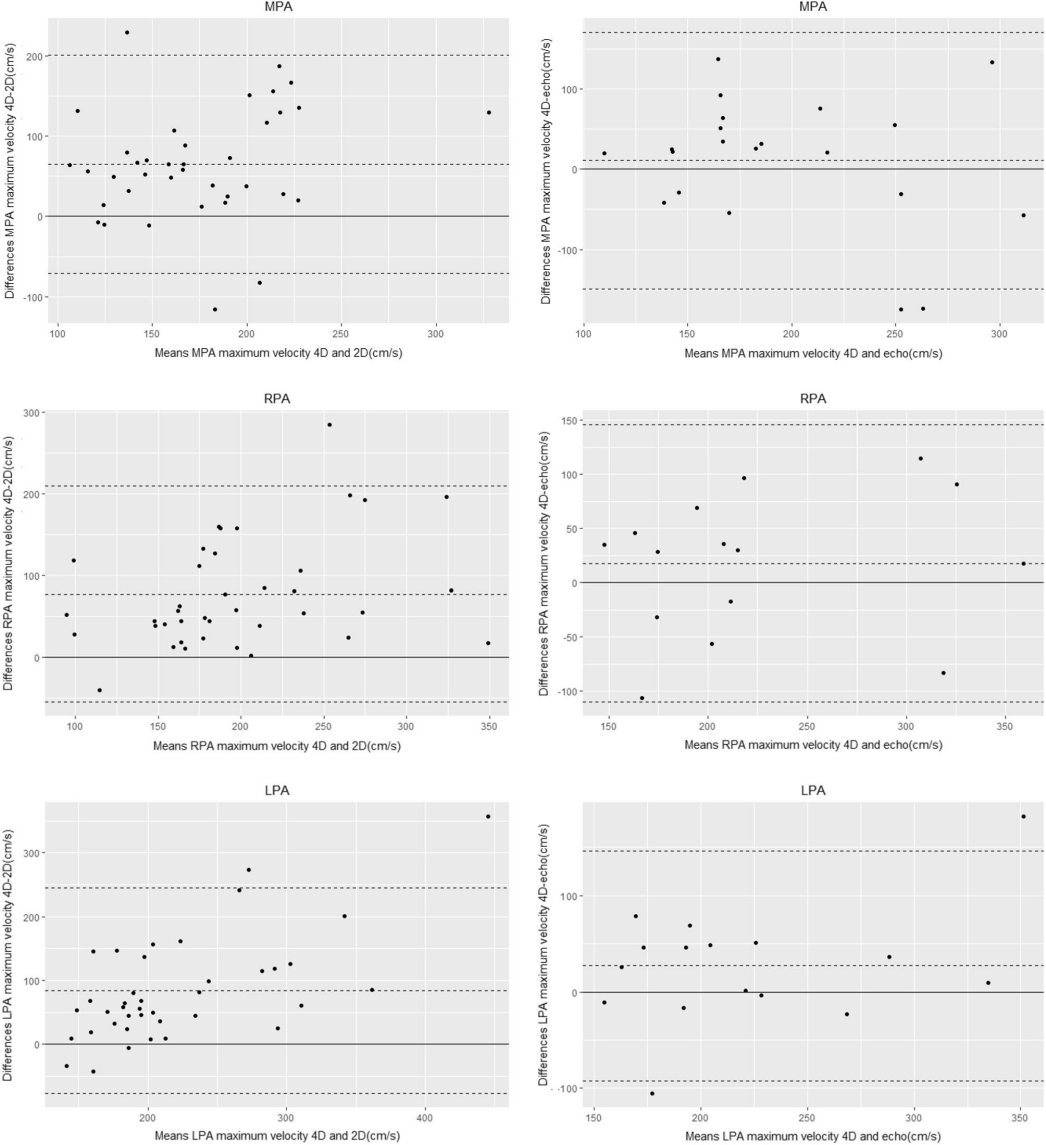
Agreement between four-dimensional flow cardiac magnetic resonance and two-dimensional phase-contrast cardiac magnetic resonance for measurement of maximum velocities in the main, right, and left pulmonary artery (left) and agreement between four-dimensional flow cardiac magnetic resonance and Doppler echocardiography of maximum velocities in the main, right, and left pulmonary artery (right). *2D* two-dimensional phase-contrast cardiac magnetic resonance, *4D* four-dimensional flow cardiac magnetic resonance, *echo* doppler echocardiography, *MPA* main pulmonary artery, *LPA* left pulmonary artery, *RPA* right pulmonary artery
